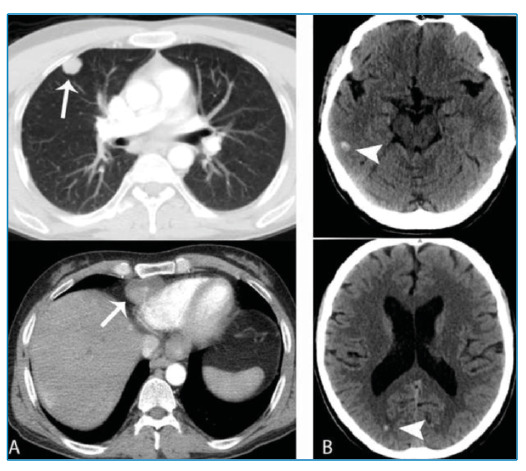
Thorax CT imaging after the transplantation (A) shows hypodense mass lesions (arrows) in the paracardiac area and within the lung parenchyma. Axial non-contrast brain CT images after the transplantation (B) show hyperdense lesions in the brain (arrowheads).
